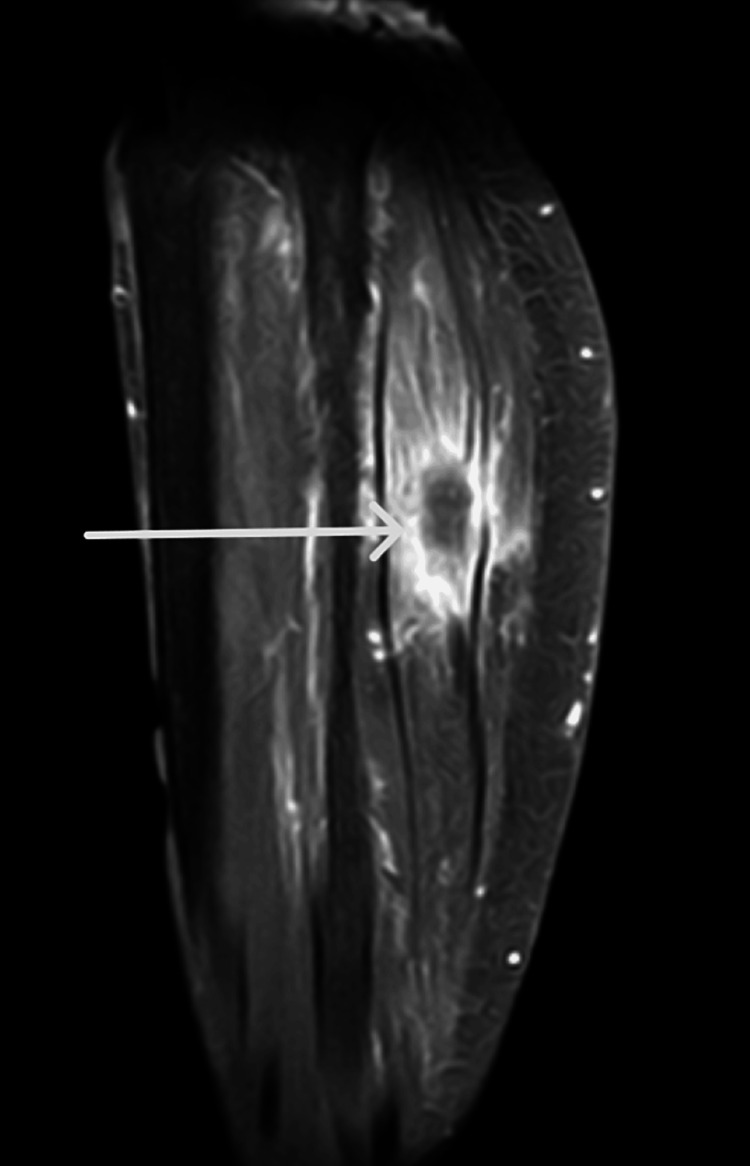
An MRI image of the right leg showing a mass (white arrow) measuring 33x33x23 mm, eroded and unevenly posterior to the fibula at the metaphyseal-diaphyseal junction
